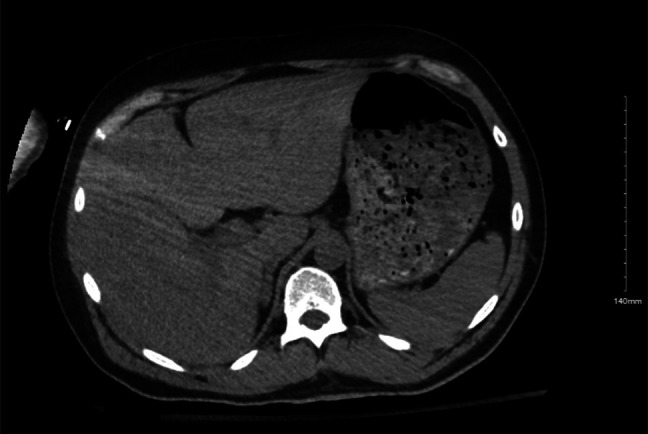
Computed tomography of the abdomen and pelvis (axial section) shows unenhanced liver without biliary duct dilation.
